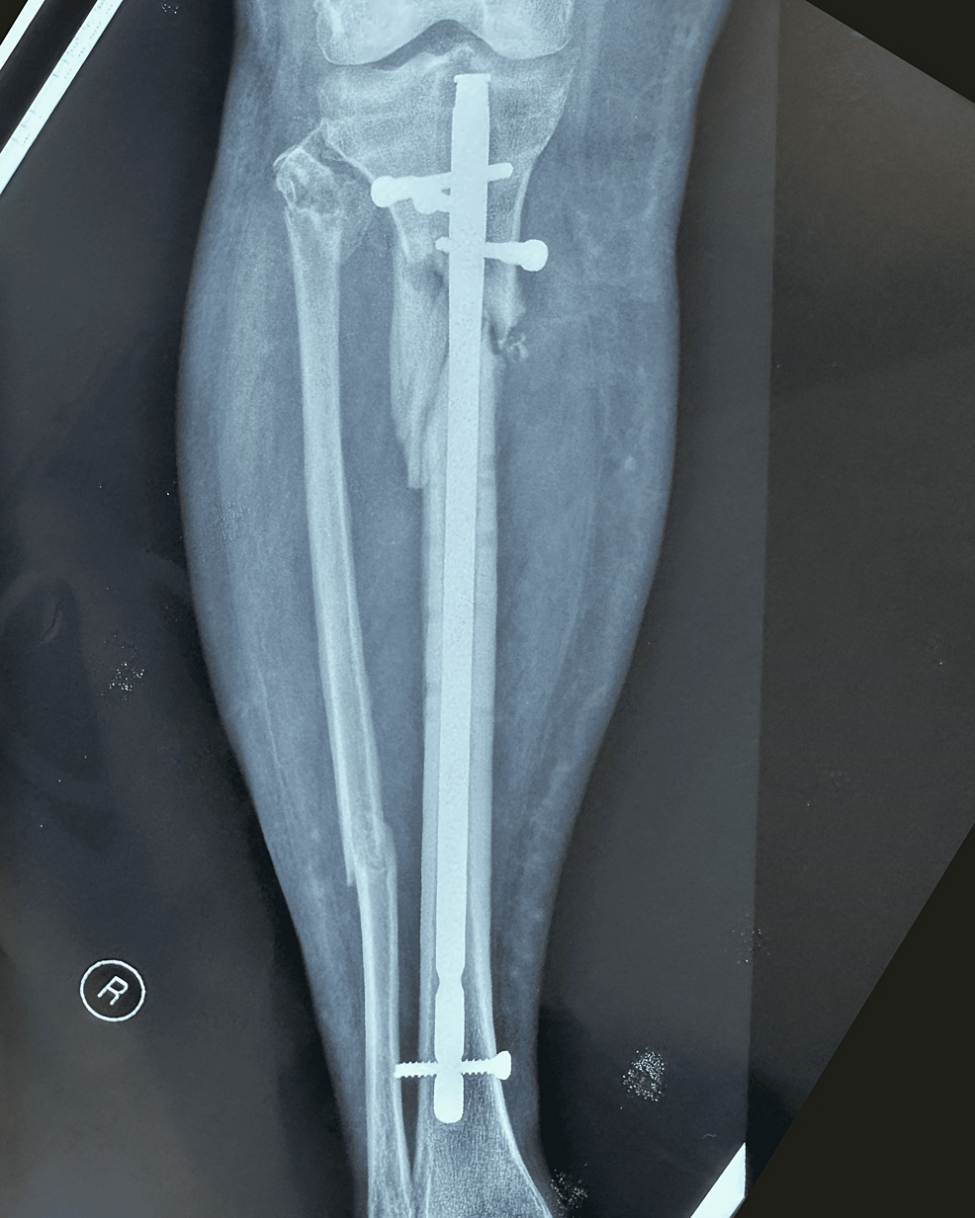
One-month postoperative anteroposterior X-ray of the right leg showing the antegrade tibial nail
